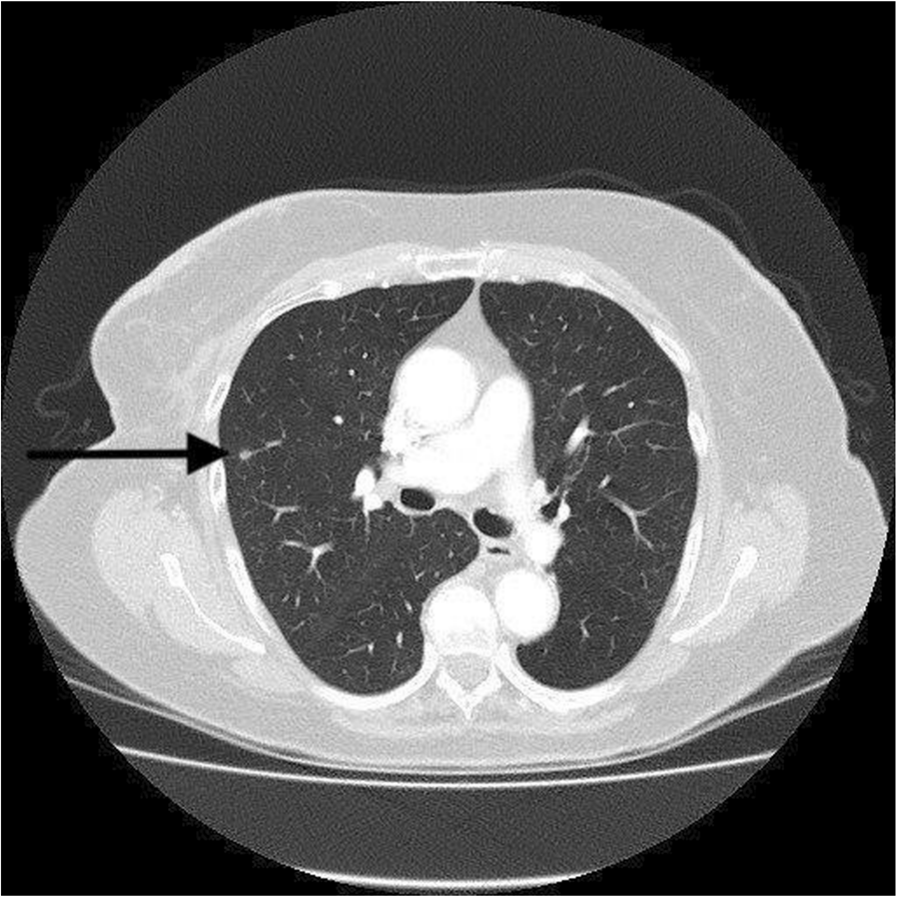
Typical indeterminate pulmonary lesion. The high sensitivity of CTC leads to the identification of very small lesions that would not be obvious on normal chest X-ray. These indeterminate results require further tests or observation to characterize the true nature of these lesions. This results in a high false-positive rate for staging CTC (10%)
